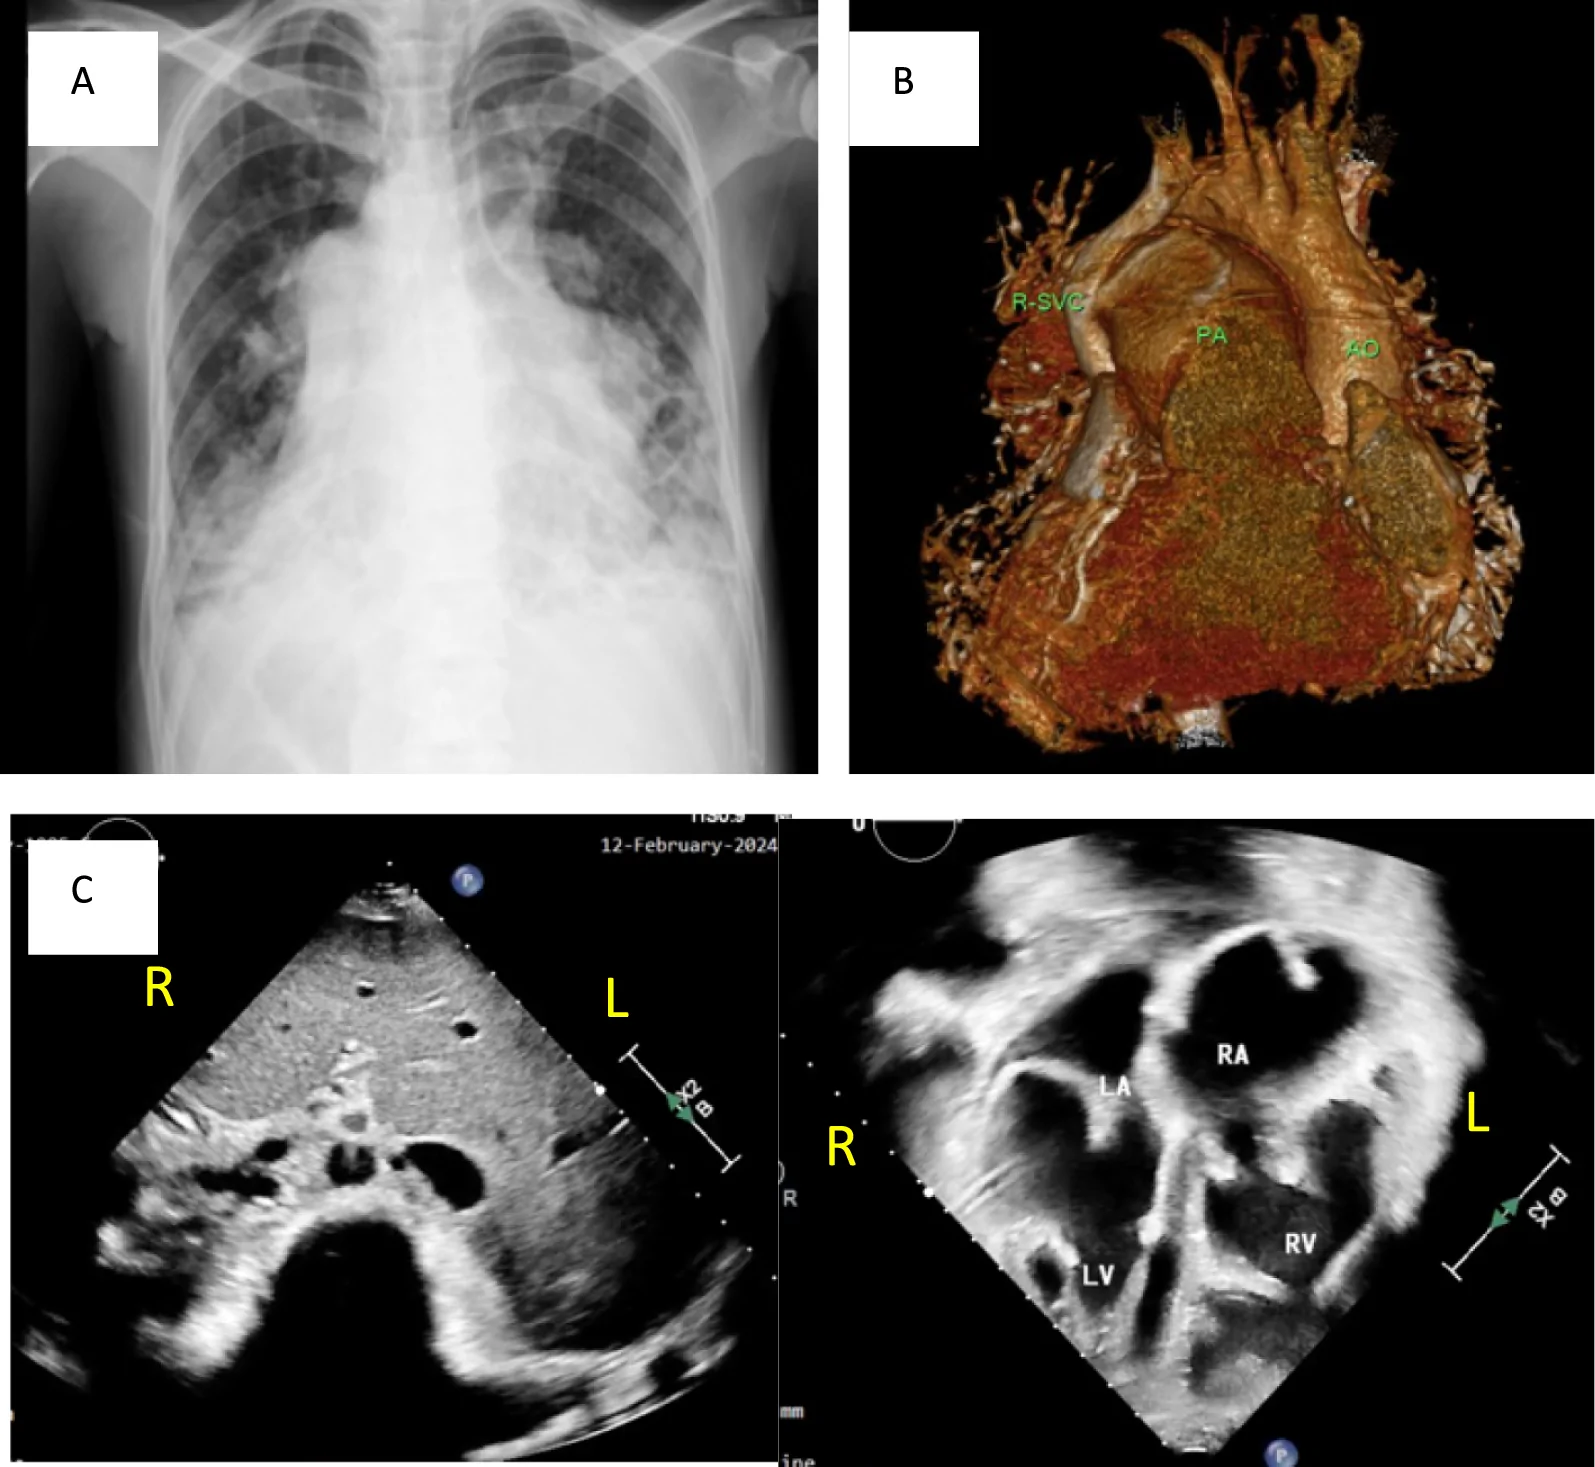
Dextrocardia, situs inversus shown in A chest X-ray; B cardiac computed tomography reconstruction; C transthoracic echocardiography
